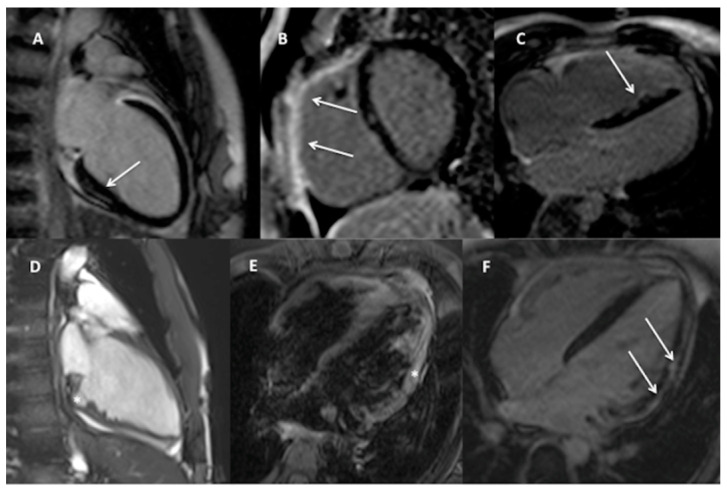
Examples of potentially arrhythmogenic CMR findings in the studied cohort: (A). Two-chamber view, non-ischemic, subepicardial area of fibrosis in the basal infero-septal segment of the LV (arrow) without signs of myocardial oedema (not shown) in a 16-year-old girl with 12%/24 h of complex LBBB-like PVCs suggestive of previous myocarditis; Band (C)—regions of LGE (arrows) in the RV outflow tract and free wall ((B)—short-axis view) and in the interventricular septum (C)—4-chamber view) in a 17-year old boy with <1%/24 h complex LBBB-like PVCs and an overall small MRI criteria for ARVC. (D). Two-chamber cine view in end-diastole showing large crypt (asterisk) and irregular myocardial pattern of the inferior LV wall in a 13-year-old boy with 14%/24 h of RBBB-like PVCs, (E,F). 4-chamber view, signs of myocardial oedema (E—asterisk) and subepicardial, non-ischemic stria of fibrosis (F—arrows) in the lateral LV wall in a 14-year-old boy with <1%/24 h of complex undetermined PVCs indicative of acute myocarditis.
